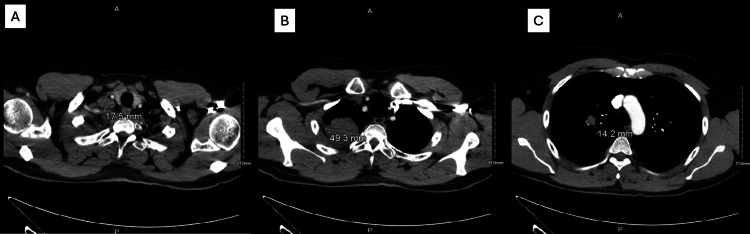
CT scan with IV contrast showing A) enlarged right supraclavicular lymph node, B) large right apical lung mass, and C) enlarged hilar masses.
